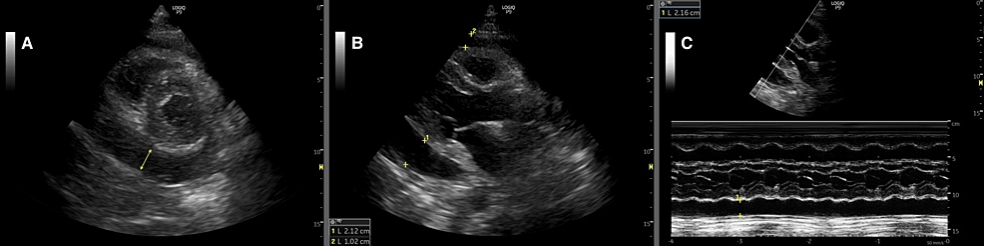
Two-dimensional echocardiogram showing features of pericardial effusion: (A) parasternal short axis view at the level of the left ventricle; (B) parasternal long axis view; and (C) parasternal long axis view motion mode at the level of the left ventricle
